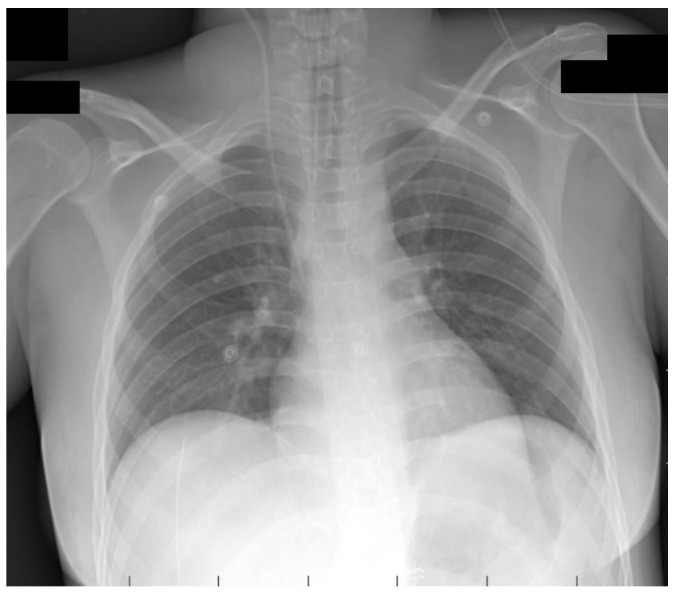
Chest X-ray seven days after surgery (anteroposterior view).
